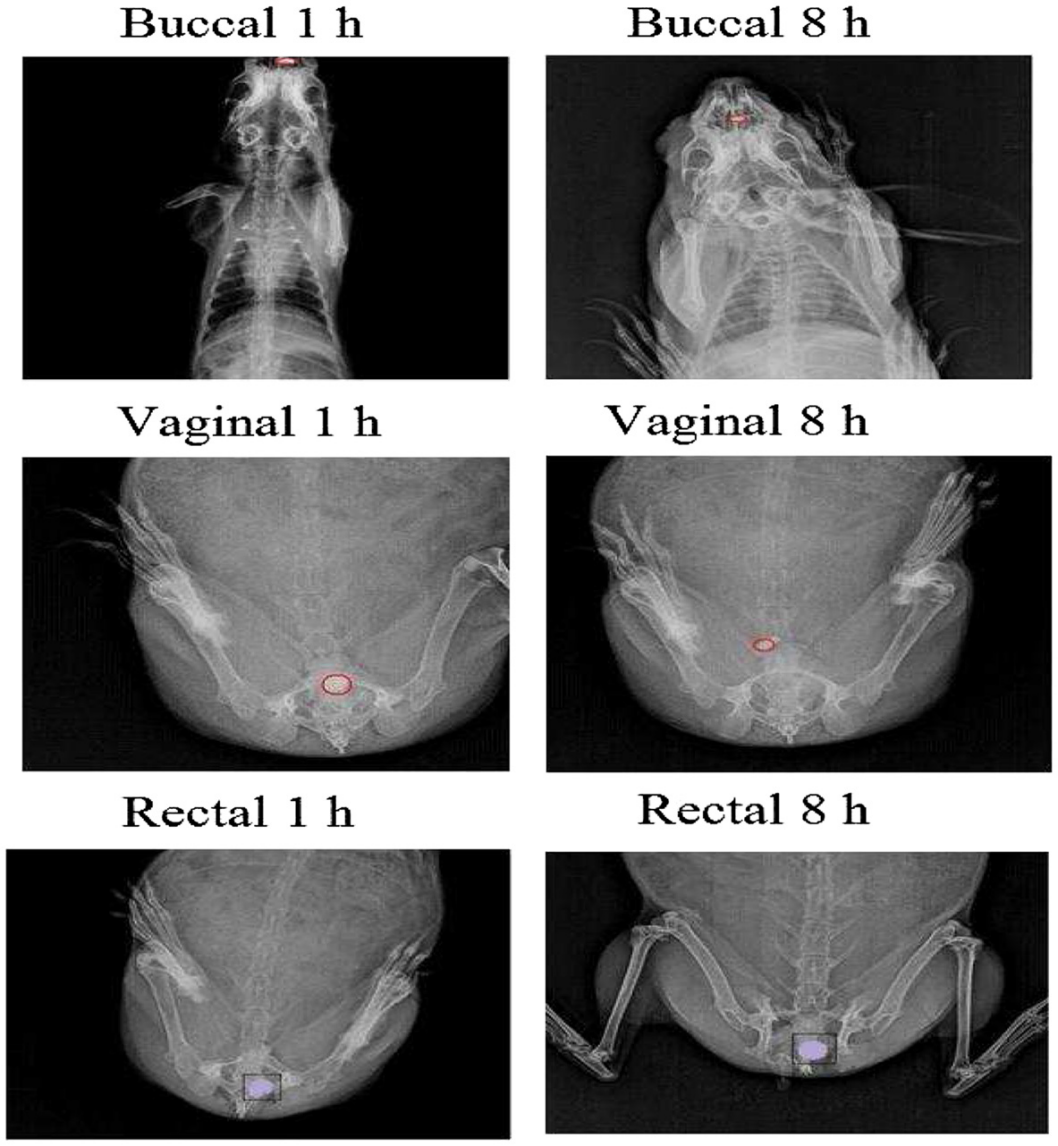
X-ray radiographic images of buccal and vaginal cavity at 1 and 8 h after ingestion of BaSO_4_-loaded optimized F4 matrix tablet.
